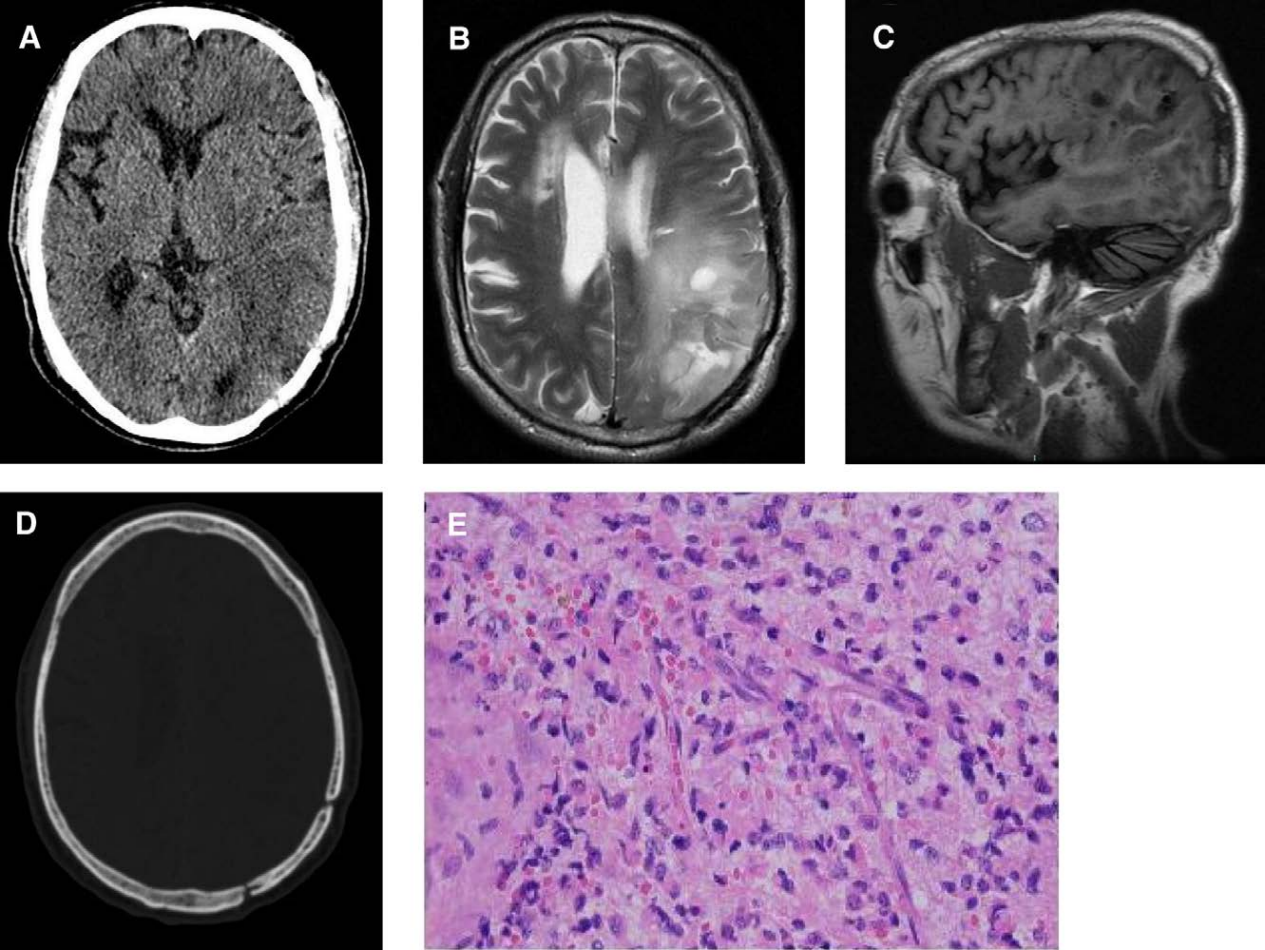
(A) Brain window, (B) (bone window) preoperative head CT showed abnormal density shadow in the left temporal occipital parietal lobe, left brain swelling, and bone discontinuity in the left occipital and parietal bones. (C) (Sagittal) and (D) (axial) preoperative MRI showing large mixed signals in the left frontotemporoparioccipital lobe, with unclear boundary, cystic degeneration, perilesional edema, and bone discontinuity in the left occipital and parietal bones. (E) Postoperative CT scan, (F) Postoperative pathological results reporting a diffuse glioma (astrocytoma) IDH mutation type, WHO III level (HE × 200), IDH = isocitrate dehydrogenase.
